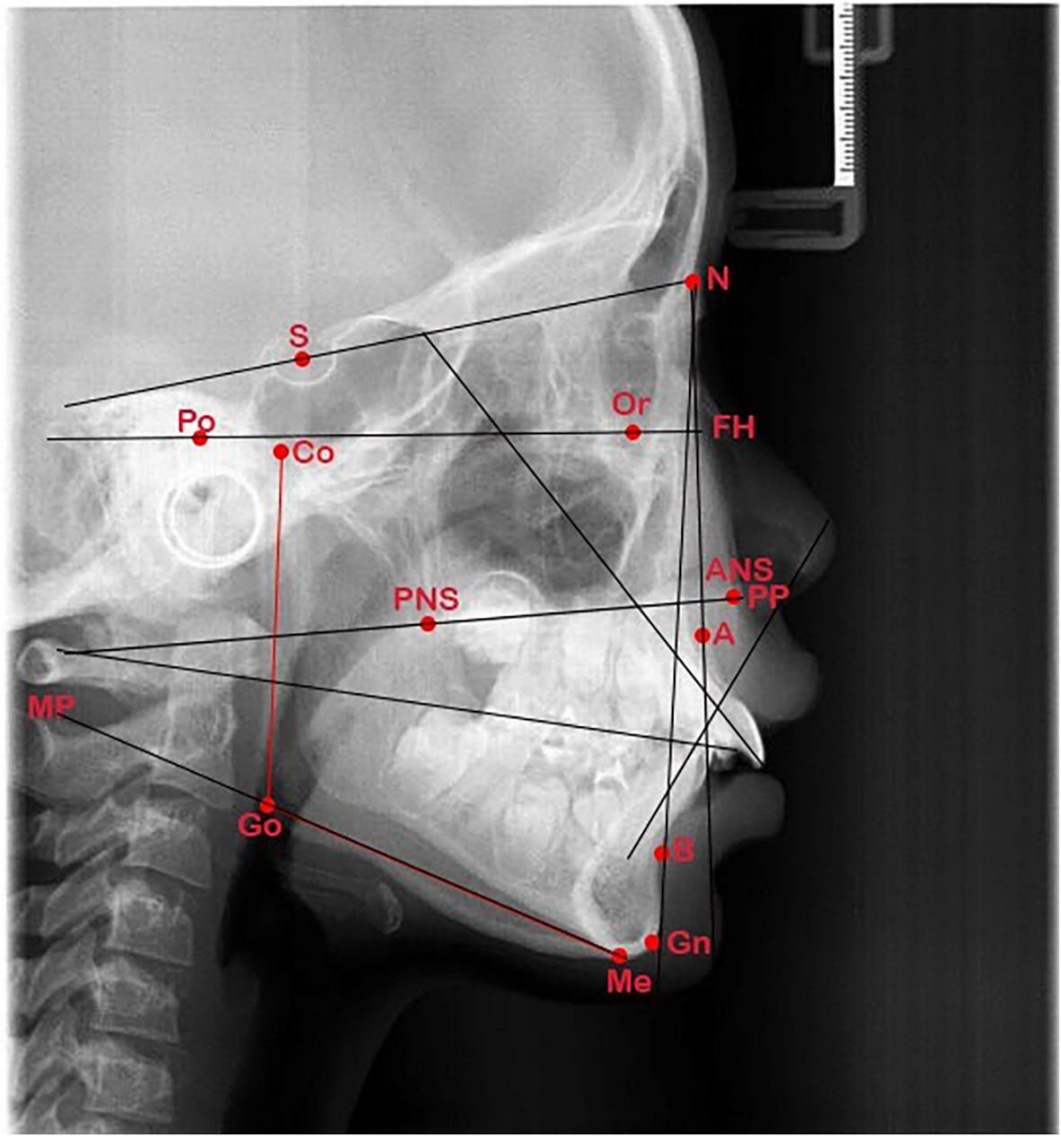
Landmarks of Cephalometric X-ray. Abbreviations of fig. 2: PNS: Posterior nasal spine, ANS: Anterior nasal spine, S: Sella, N: nasion, A: A-point, B: B-point, GO: Gonion, GN: Gnathion, Me: Menton, Po: Porion, Or: Orbitale, CO: condylion FH: Frankfort horizontal plane, PP: Palatal plane, MP: Mandibular plane, OP: Occlusal
